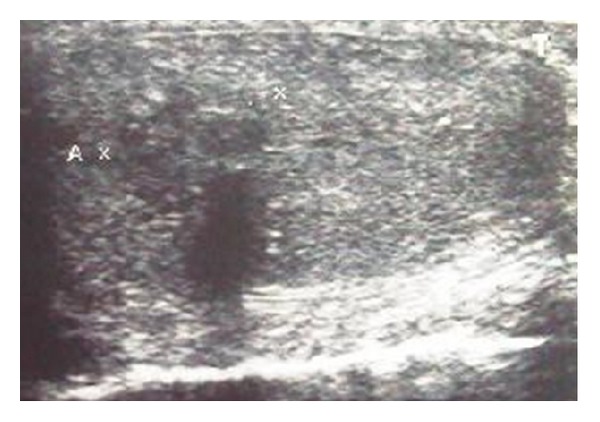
Testicular ultrasonography. Multiple hypoechoic areas in the testes. Both epididymides were normal.
